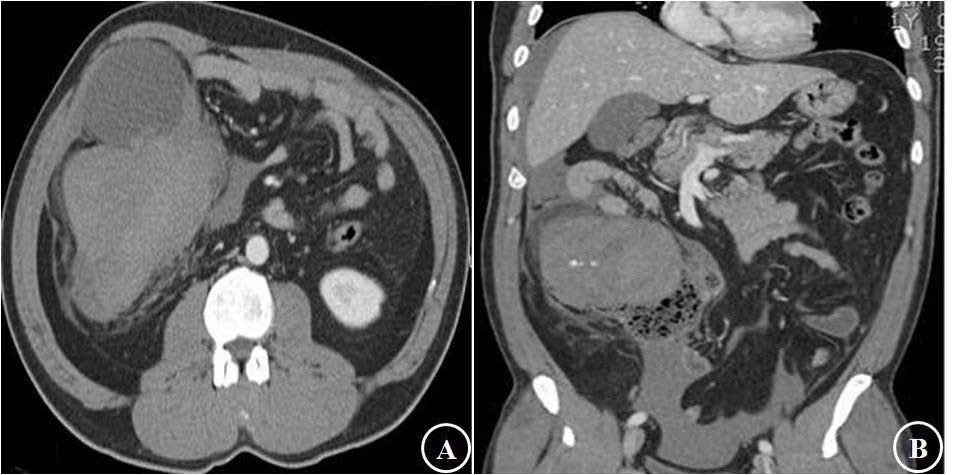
Preoperative computed tomography(CT) findings. A: a 15 × 10 cm sized solid mass with cystic lesion which was located in ascending colon, B: the mass was slightly enhanced and some of high density fluid collections in pelvic cavity.
